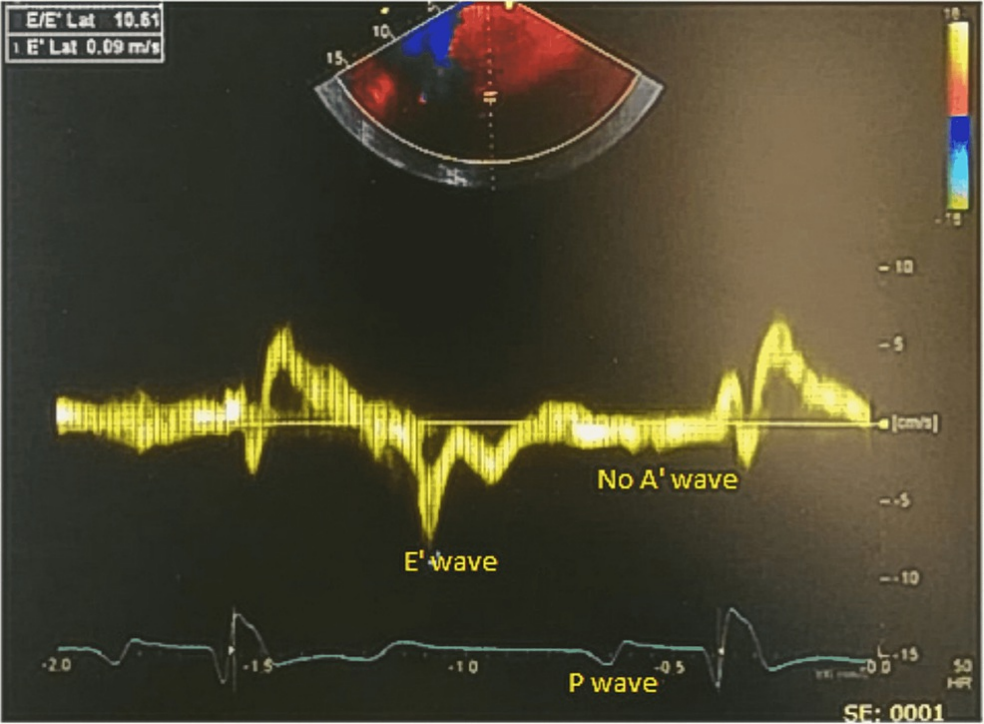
Tissue Doppler showing the presence of E’ wave and P wave but the absence of A’ wave
